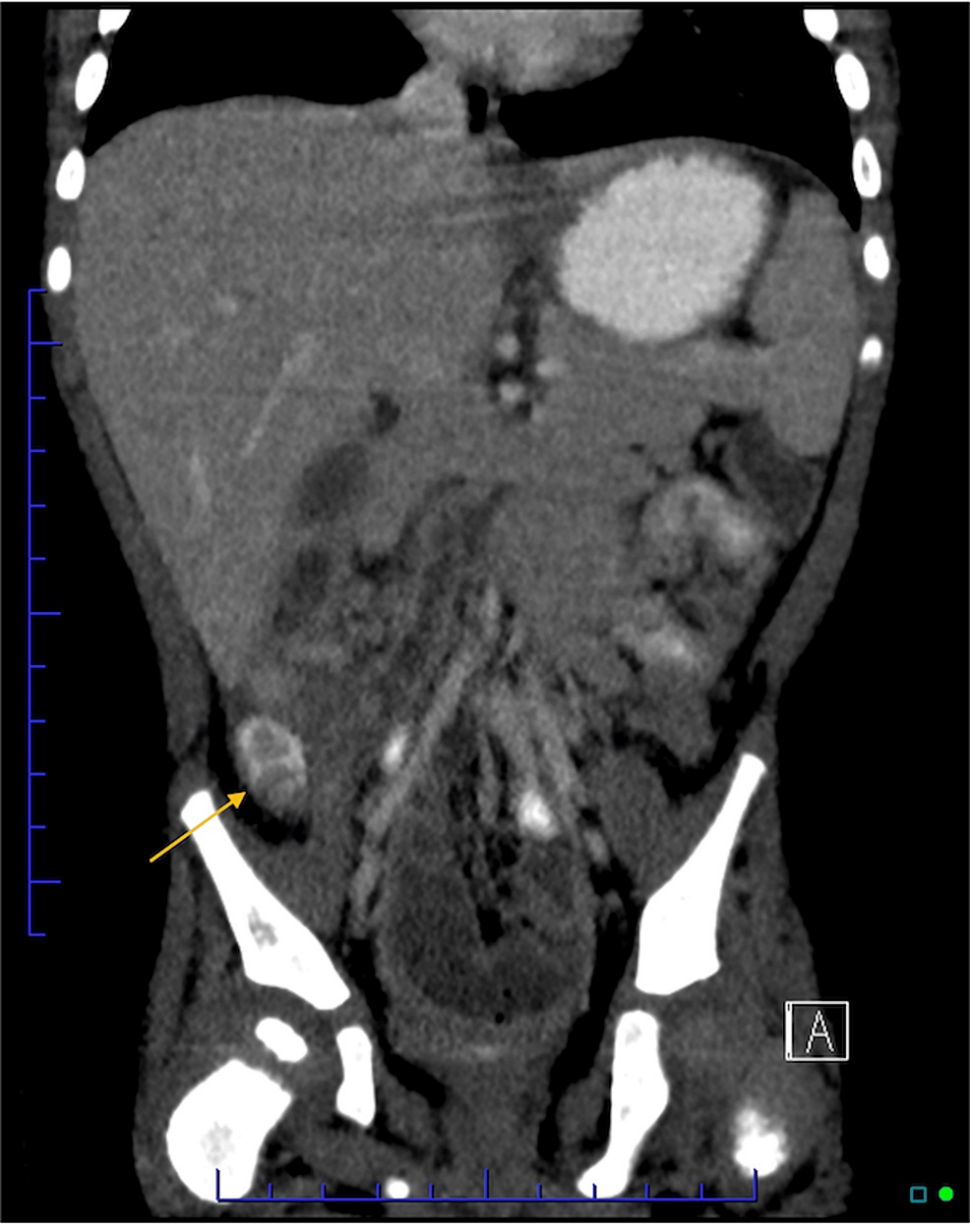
CT Abdomen/pelvis with IV and oral contrast: Demonstrating a fluid-filled tubular structure, prominent in size, in the right hemiabdomen likely to be retro-ileal appendix with adjacent inflammation/fluid, favored to represent appendicitis (coronal section)
